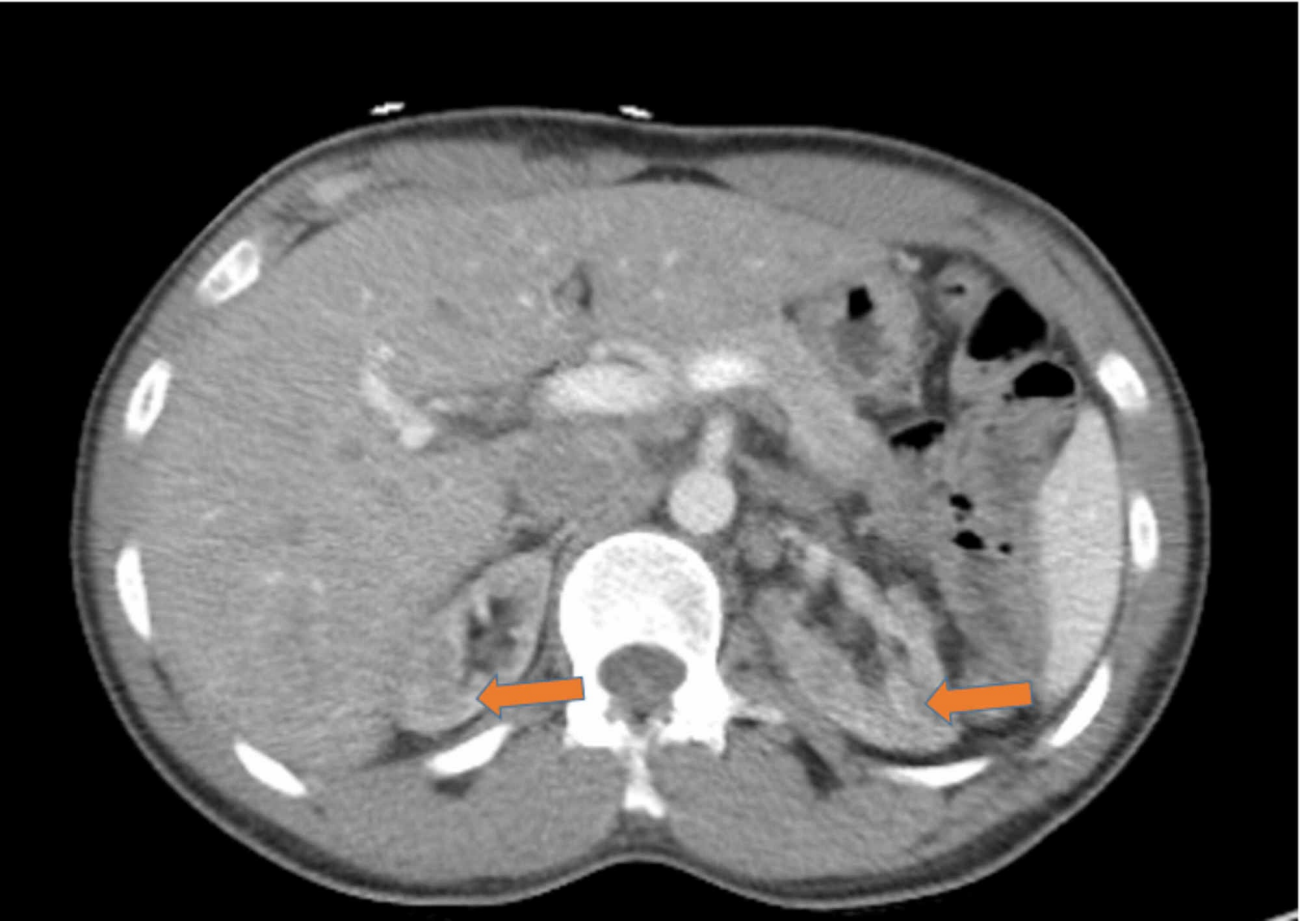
CT of the abdomen and pelvis without intravenous contrast in an axial view showing bilateral renal atrophy, without any evidence of hydronephrosis, pyelonephritis, renal mass, or vascular abnormality. CT, computed tomography
